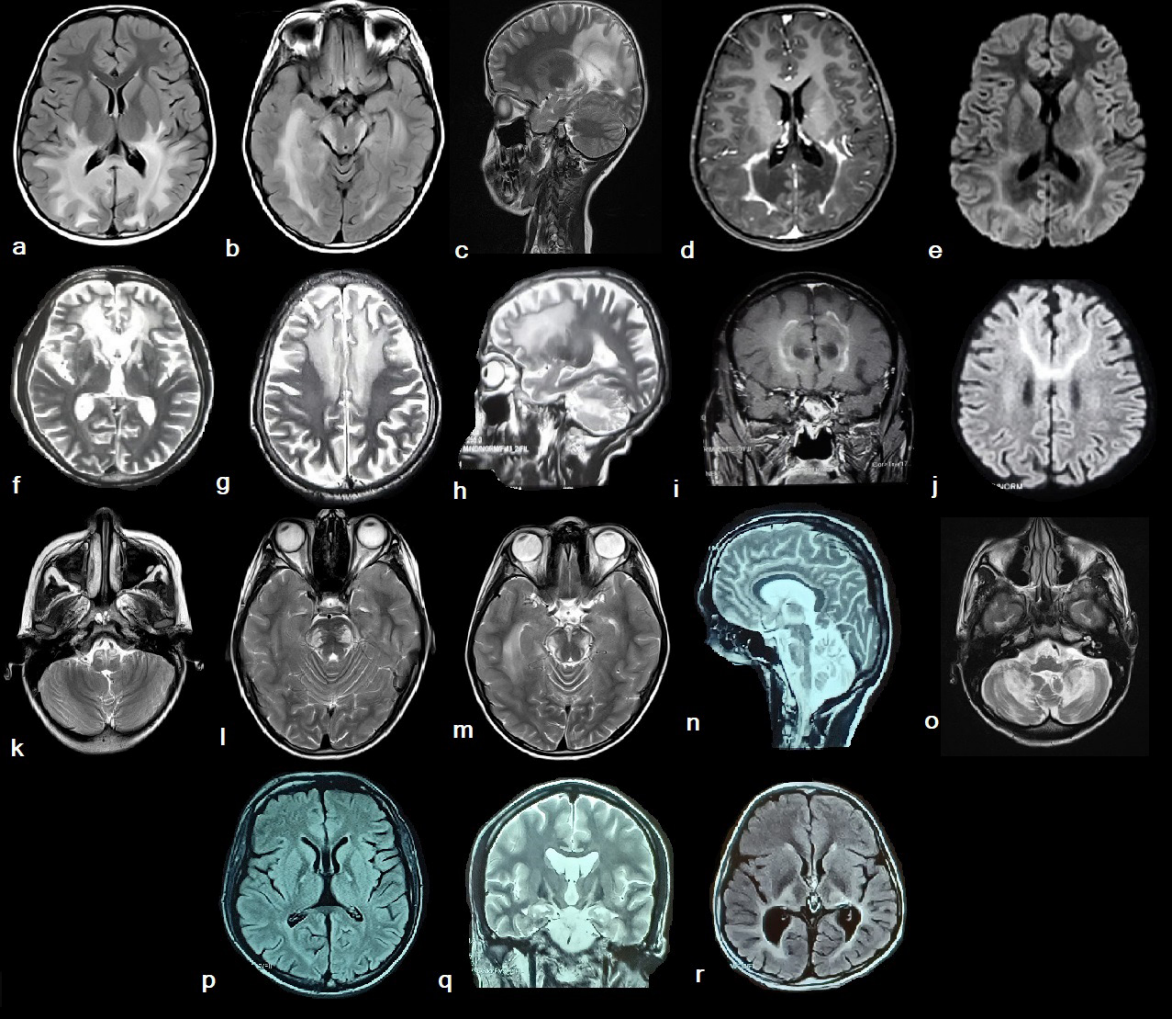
Radiological profile of patients with ALD on magnetic resonance imaging of the brain including T2, T2 Flair, contrast, and DWI sequences. Row 1: (a–e) Parieto-occipital pattern of ALD with involvement of parieto-occipital white matter (a, c), occipito-temporal white matter (b), and splenium of corpus callosum (a), with contrast enhancement (d), and DWI changes (e). Row 2: (f–j) Frontal pattern of ALD with involvement of frontal white matter (f, g, h), genu of corpus callosum (f), with contrast enhancement (i), and DWI changes, “stag horn appearance” (j). Row 3: (k–o) Posterior fossa involvement in ALD with hyperintensity along corticospinal tracts in medulla (k), pons (l), and midbrain (m), cerebellar atrophy (n), and cerebellar hyperintensities (o). Row 4: (p–r) White matter hyperintensity noted along bilateral corticospinal tracts (p, q) with a “wine glass appearance” on coronal view (q). Bilateral thalamic involvement in ALD (r).
